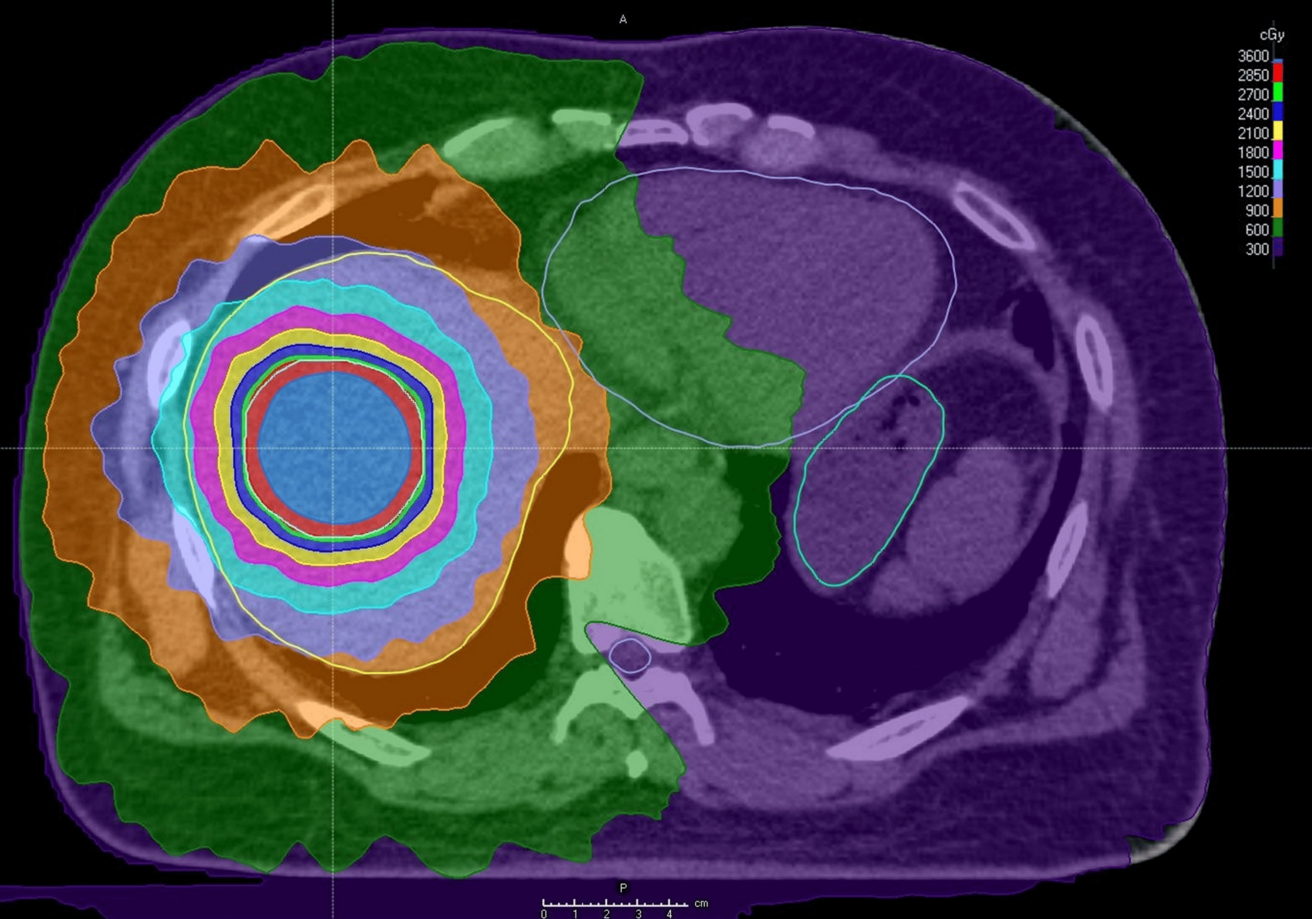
Treatment plan overlaid on a CT scan of the abdomen. Stereotactic body radiotherapy treatment plan with the prescribed radiation dose shown in the upper right region. The area colored in red represents the region where at least 95% of the prescribed dose is delivered, and the tumor is located within this area. Organs at Risk (OARs): the stomach is outlined in sky blue, the heart in lavender, and the liver in yellow. The outlines around the organs highlight these OARs.
